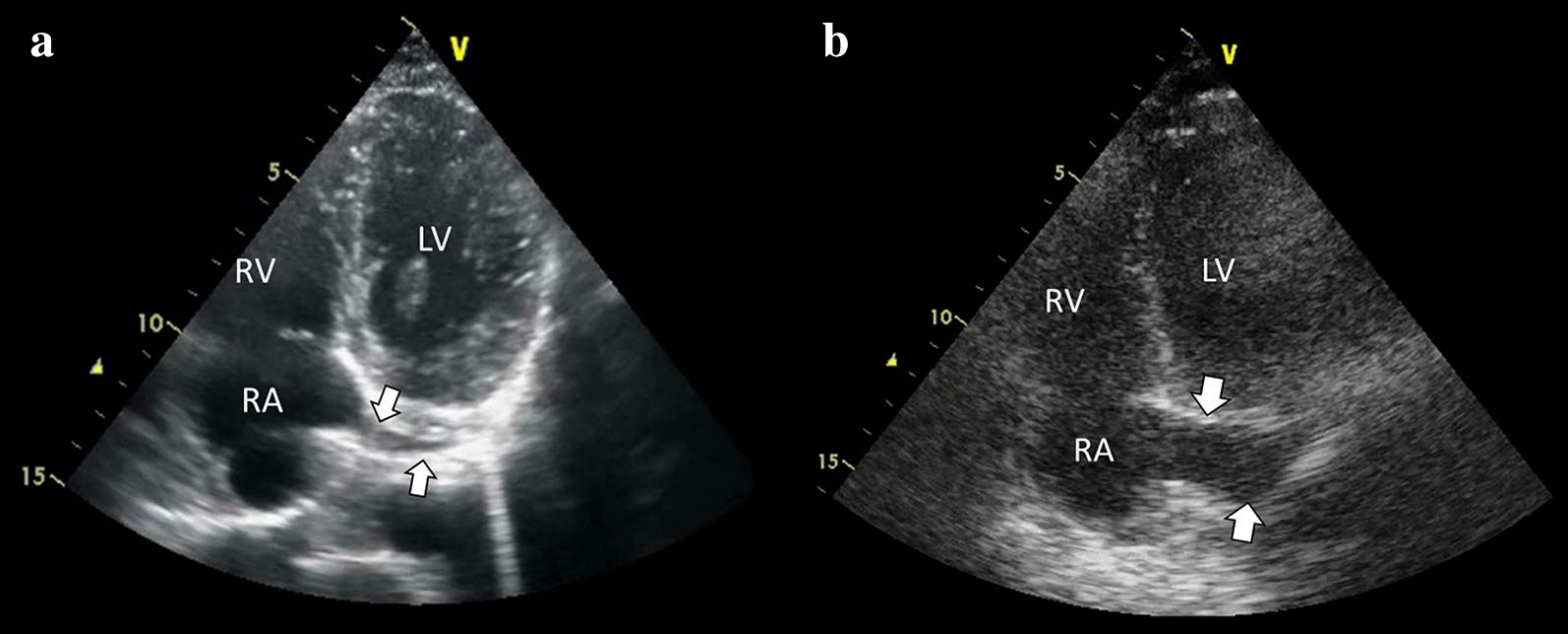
Coronary sinus view, obtained from a foreshortened apical 4-chamber view. a Normal coronary sinus (arrow). b Dilated coronary sinus (arrows)
